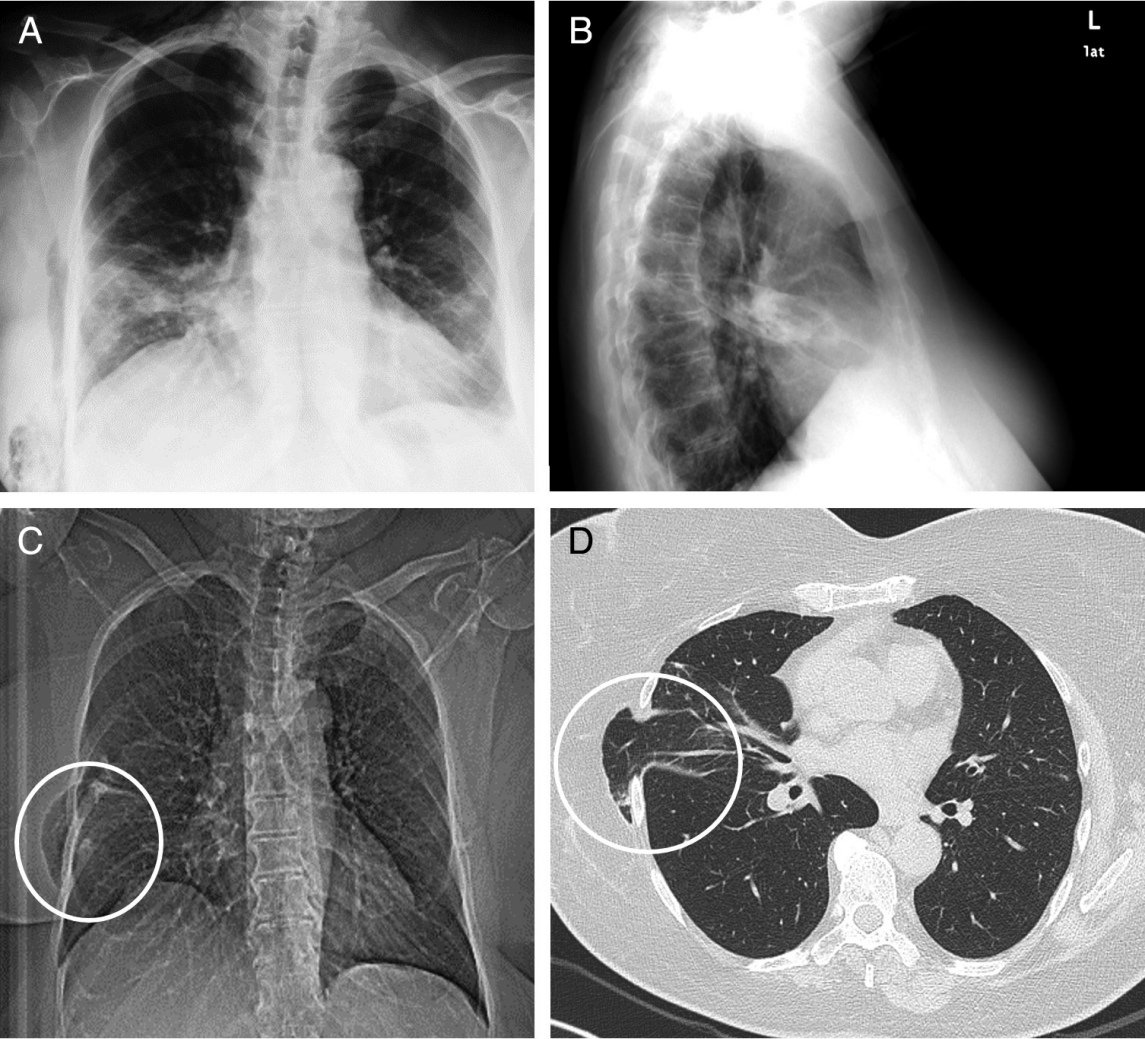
Chest X‐ray after nodulectomy surgery: subcutaneous, right, parietal emphysema (A and B). Chest computed tomographic (CT) scan showing lung hernia (C and D, white circles).
